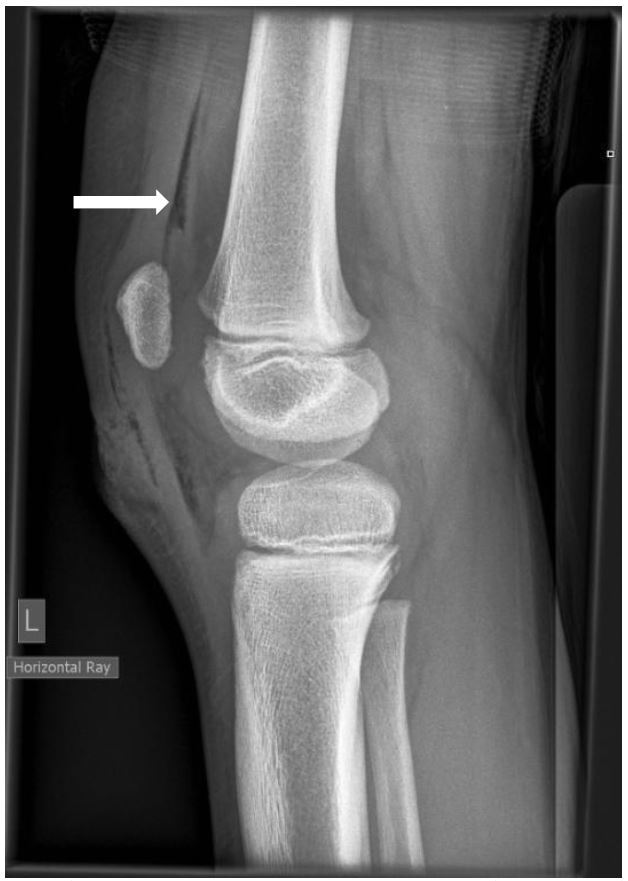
Lateral radiograph of knee showing gas in the suprapatellar pouch of the joint capsule.
